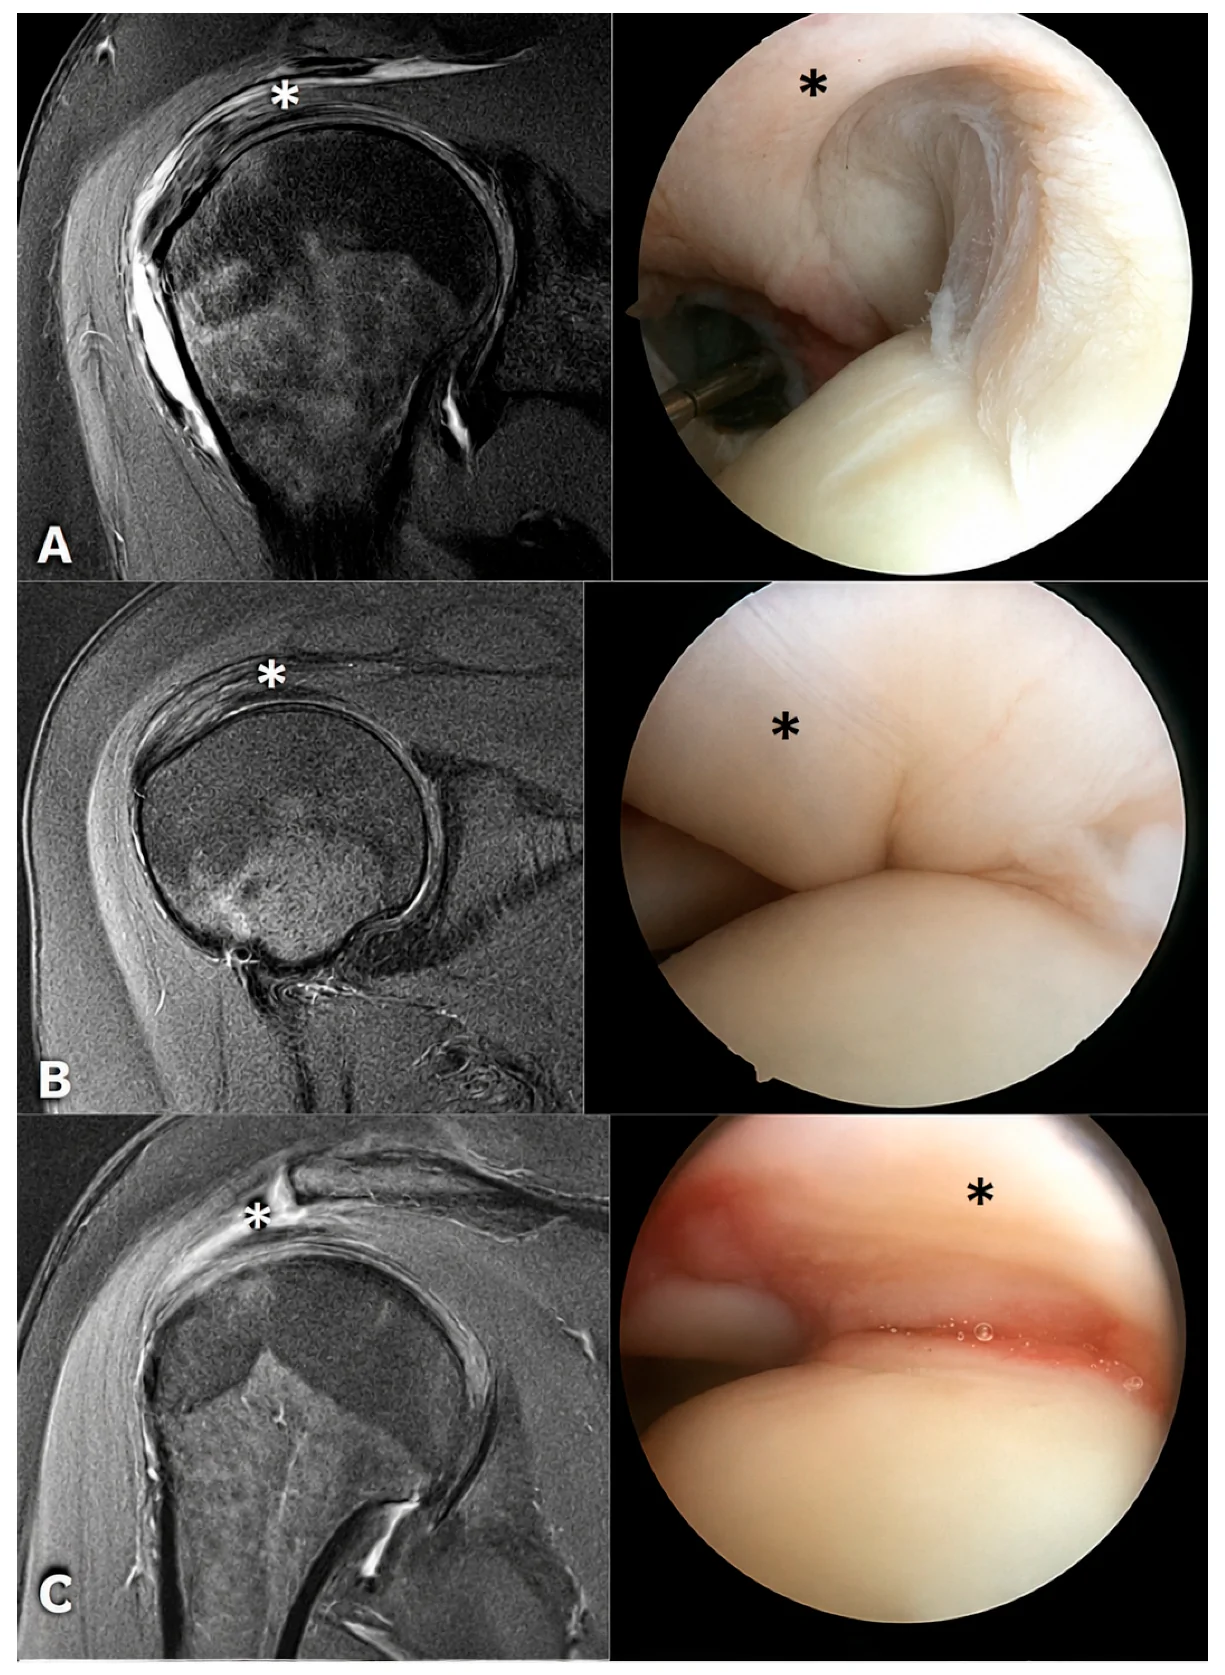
Representative MRI ((left), oblique coronal view) and corresponding arthroscopic (right) images from three different patients with right shoulders in whom the rotator cable was identifiable on both MRI and arthroscopy. (A–C) Representative MRI and corresponding arthroscopic images from three different patients. Each letter (A, B, and C) represents a different patient. * indicates the rotator cable.
